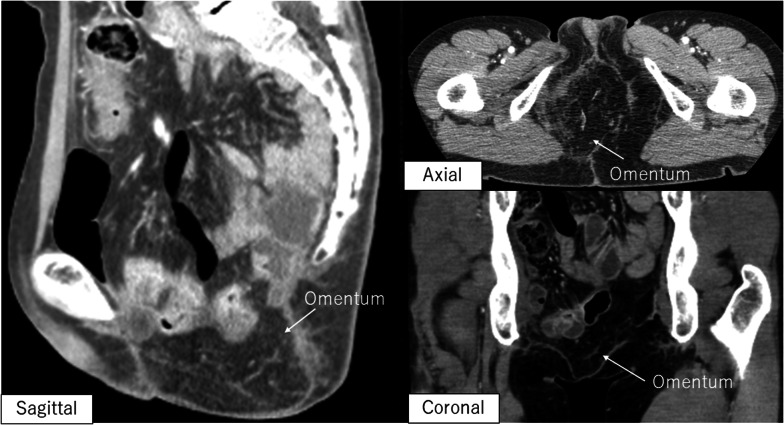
Postoperative computed tomography scan. The dead space in the ischiorectal fossa is filled with omentum. No perineal hernia is evident
